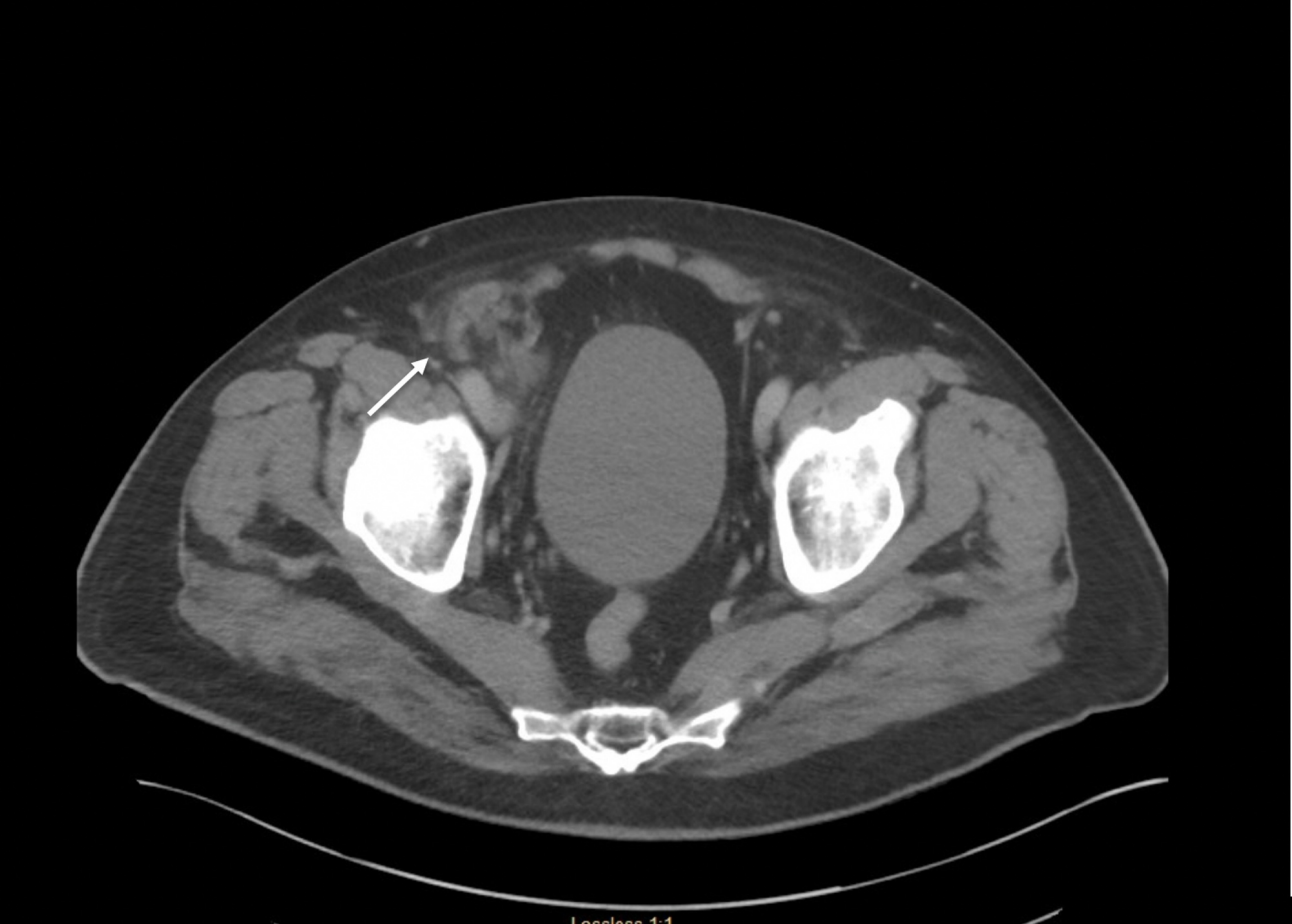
CT scan of the abdomen and pelvis revealing an incarcerated right femoral hernia with an inflamed appendix CT = computed tomography
